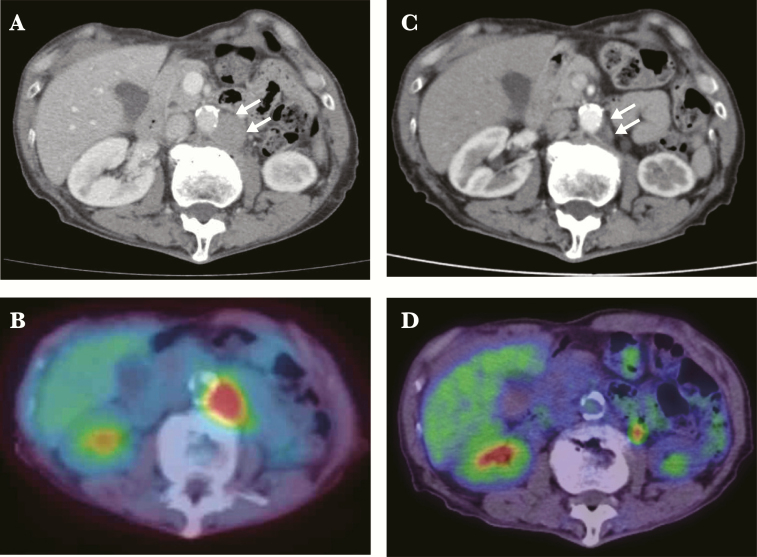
Representative images of B-cell lymphomas before and after direct-acting antiviral agent (DAA) treatment against hepatitis C virus (HCV). Images before antiviral treatment (A and B) and after sustained virologic response (C and D). Para-aortic lymph node detected by computed tomography (CT) (A and C) and detected by positron emission tomography using the glucose analog ^18^F-fluorodeoxyglucose (^18^F-FDG-PET) with CT (FDG-PET/CT) (B and D). White arrow indicates the lymphoma. The FDG-PET/CT detected diffuse high uptakes in the para-aortic lymph node (B).
